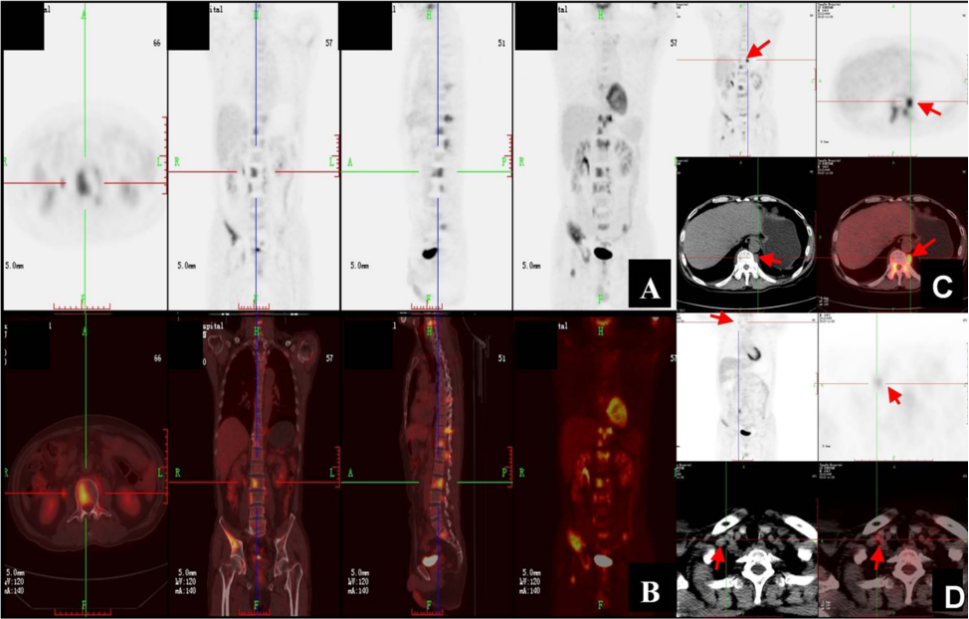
Representative whole body PET-CT scans. (A) and (B) Multiple foci of increased 18 F-fluoro-2-deoxyglucose (FDG) uptake at the bilateral iliac bones, C4, T7, T11, T10, T12, L2, L3, S1 vertebral bodies and the right acetabulum; (C) Focus of increased ^18^ F-FDG uptake at the lymph node near the left side of the T11 vertebral body (arrows); (D) Focus of increased ^18^ F-FDG uptake at the lymph node of the right supraclavicular region (arrows).
